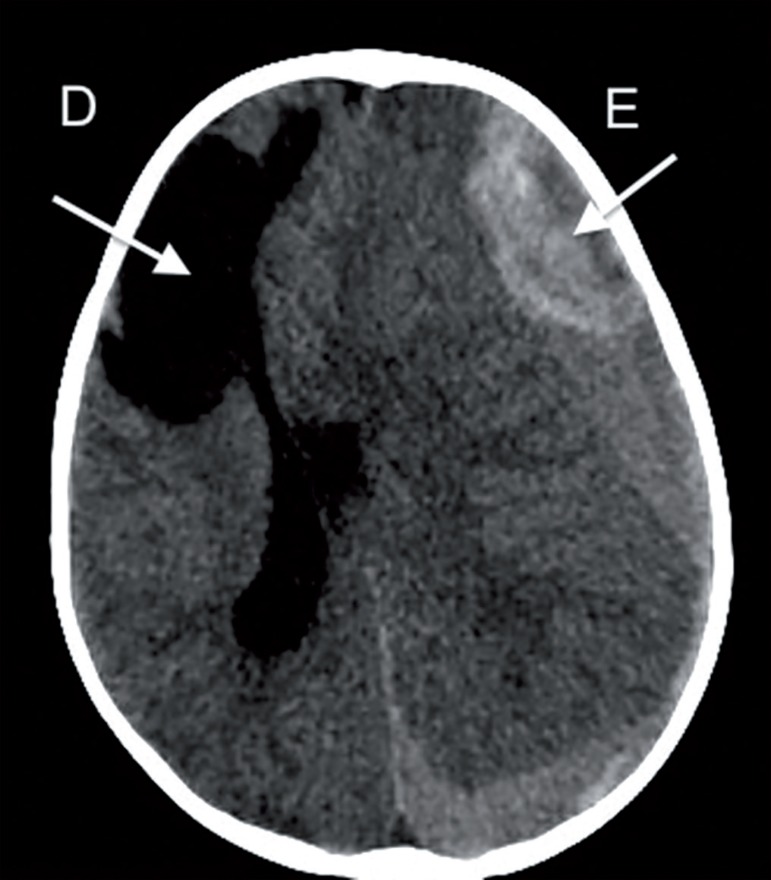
Initial computed tomography scan of the skull: evidence of a brain
